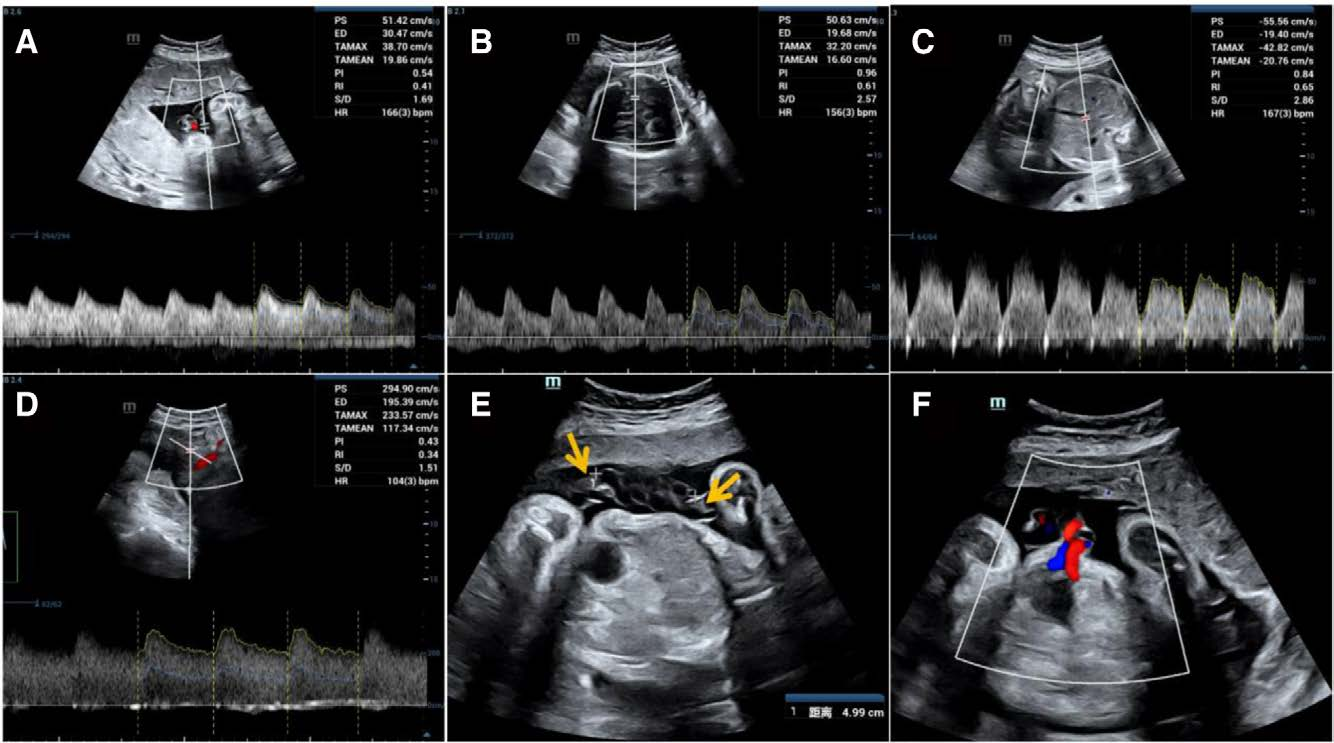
Hemodynamics arrangement of the UAT fetal. The values of UA-PI, RI, and S/D (A), MCA-PI (B), left UtA PI (C) were all <5th centile for gestational age of 32 weeks. The value of systolic/atrial wave ratio of ductus venosus (D) was in the 50th to 95th centile for gestational age of 32 weeks. (E) The excessive torsion of UC (the umbilical coiling index was 3/4.99 = 0.6) and 2 hypoechoic thrombosis (orange arrows) were observed through 2-dimensional ultrasound. (F) Only 1 UA showed in the section of bladder through color Doppler ultrasound. MCA = middle cerebral artery, PI = pulsatility index, RI = resistance index, S/D = systolic/diastolic, UA = umbilical artery, UAT = umbilical artery thrombosis, UC = umbilical cord, UtA = uterine artery.
